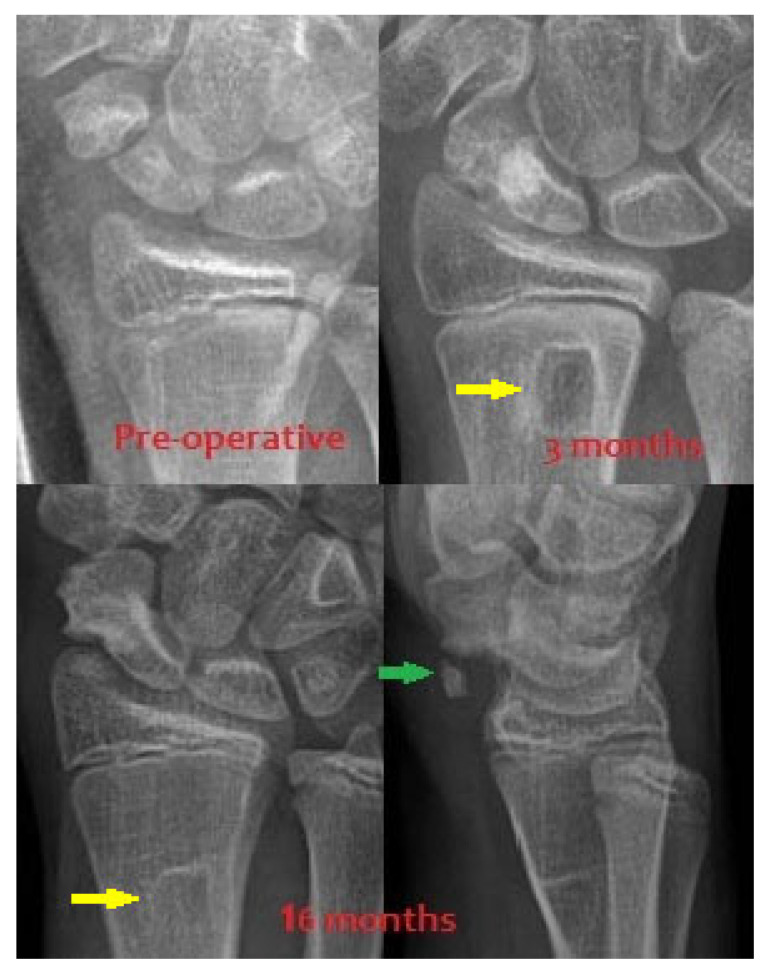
Scaphoid healing process in Patient 1. Top row: Preoperative and 3-month postoperative anteroposterior (AP) projection X-rays. Bottom row: X-rays at 16 months postoperatively. The lateral view shows the ossified pedicle of the vascularized graft (*green arrow*). It causes neither discomfort nor functional impairment and may be surgically removed during the K-wire extraction at the surgeon’s discretion. Also visible is the proximal shift of the graft harvest site due to distal radius growth, indicating that no epiphysiodesis occurred (*yellow arrow*).
